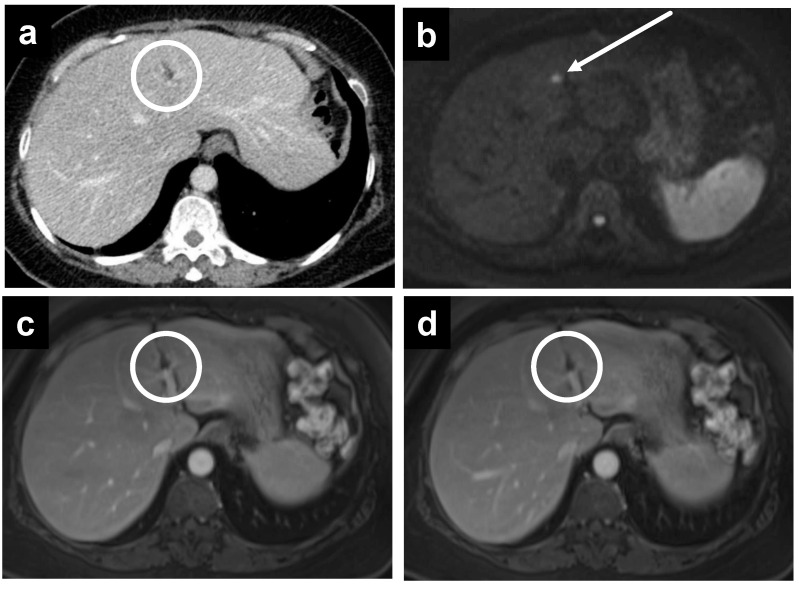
A 61-year-old female with subtle recurrent primary peritoneal carcinoma, a tiny deposit is only well seen on the (b) DWI as a hyperintense focus at the falciform ligament (white arrow). A rim-enhancing nodule is barely seen on (a) CT, as well as the (c) portal venous and (d) delayed phases on MRI (white circles).
